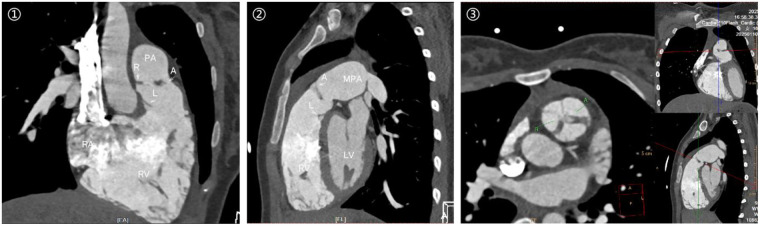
Cardiac computed tomography angiography demonstrating aneurysmal dilatation of the main pulmonary artery (diameter 44 mm, arrow) and marked right ventricular enlargement.
